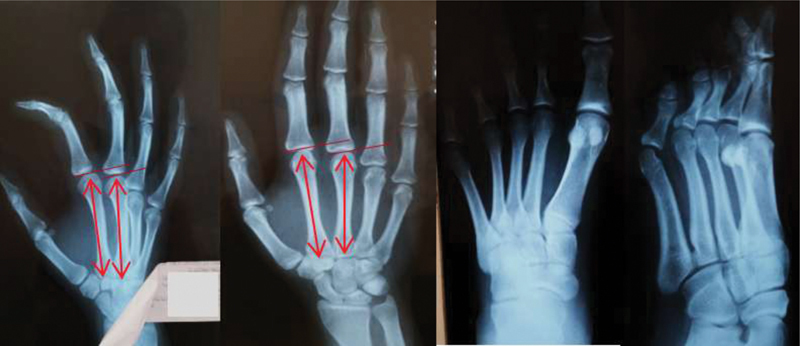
Case 1: radiographs of the right hand (normal) and left foot (ipsilateral to the lesion) to determine the length of the second metacarpal (MC) and its relationship with the third MC, in addition to verifying which metatarsus presented greater anatomical similarity to the second MC.
